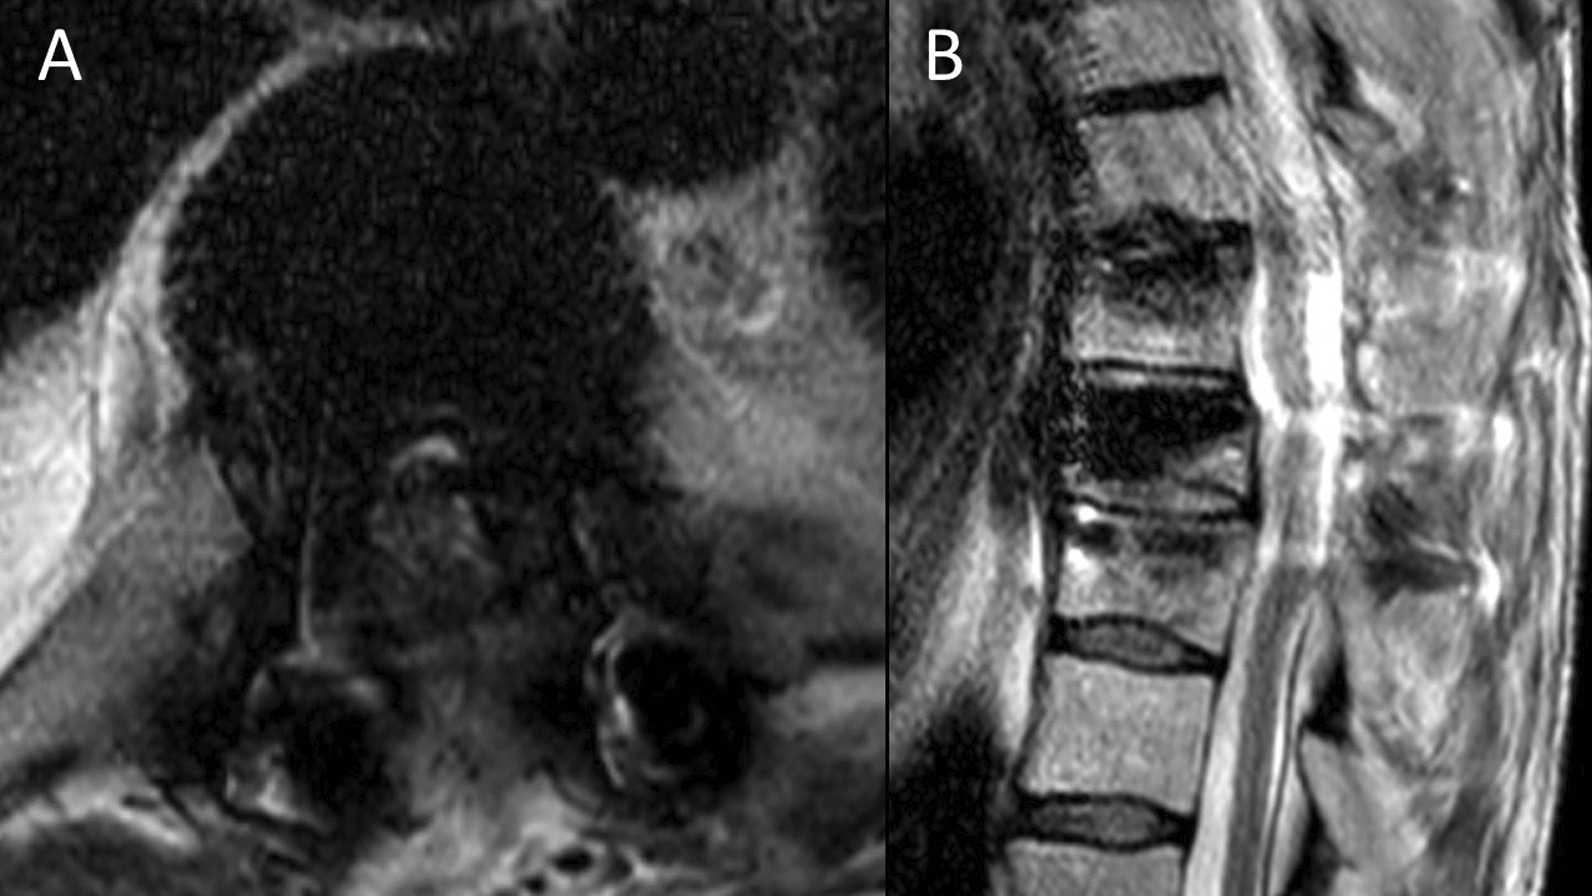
Dorsal spine T2-sequence magnetic resonance imaging showing the kyphoplasty at T8 level with posterior fixation at T7 and T9. A axial view showing intracanal cement leakage at T8 level, without visible compressive consequences on the dorsal spinal cord. B sagittal view showing no myelopathic consequences at T7-T9 level
